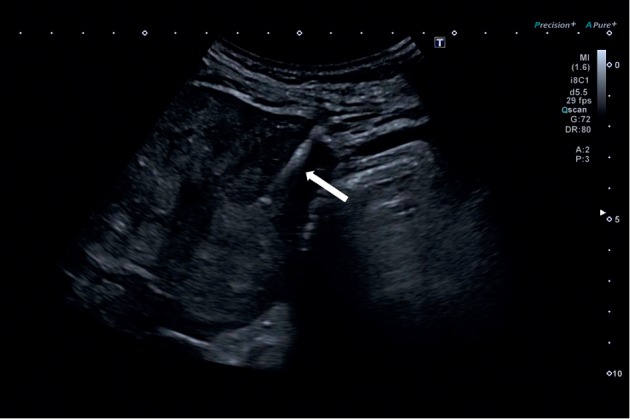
Ultrasound-guided percutaneous biopsies of the lesion located in the liver segments II-III. White arrow shows the needle penetrating in the subglissonian isoechoic/hypoechoic solid nodule with irregular margins (maximum diameter: 2.2 cm).
